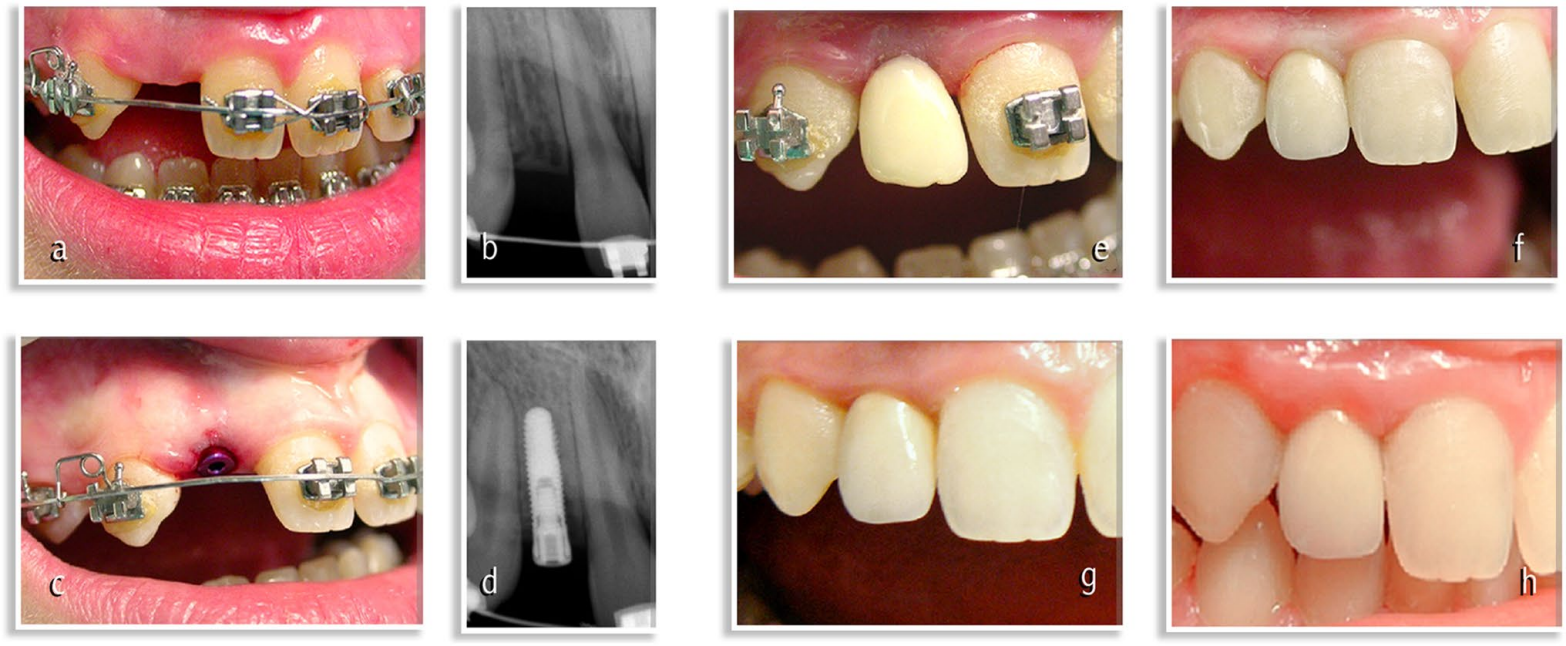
a Maxillary lateral incisor agenesis: orthodontic opening of the space in regions 1.2. b Radiographic evaluation of alveolar crest in region 1.2. c Post-surgical implant sites in regions 1.2 and 2.2. d Radiographic evaluation post-surgical implant site in region 1.2. e Peri-implant tissue aspect after 1 month. f Peri-implant tissue aspect after 1 year. g Peri-implant tissue aspect after 5 years. h Peri-implant tissue aspect after 10 years.
